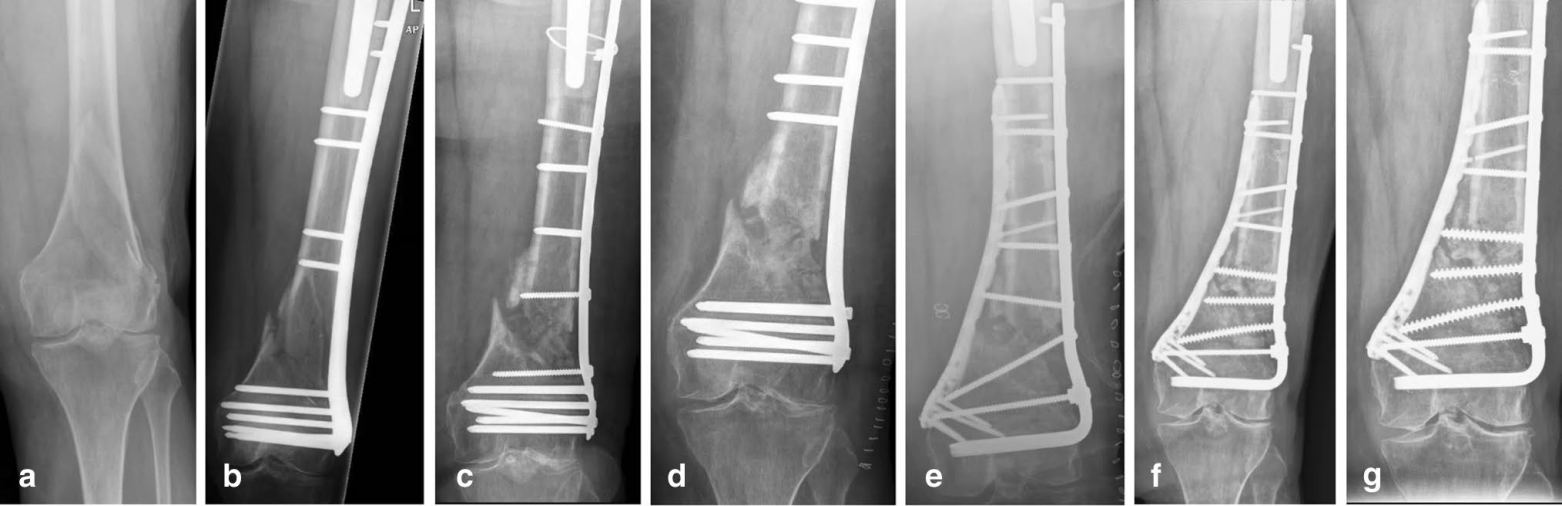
A seventy-six year old female was referred to us after several attempts to heal a comminuted extra-articular distal femur fracture (33A3) (a). Initial fracture treatment in another institution consisted of a LISS plate (b). Revision surgery with a new LISS plate and cerclage around hip prosthesis was performed 8 months after initial fracture treatment (c). Three days later a second revision surgery with a LISS plate was performed due to malposition of the previous plate (d). Six months later the patient underwent additional bone grafting surgery and was then referred to us with a persistent nonunion. At 20 months after the initial fracture we performed our index nonunion surgery with a 95-degree condylar blade plate and a Philos plate medially. In addition, autologous bone grafting was performed (e). Another bone grafting surgery with addition of three cancellous screws was performed six months later. Union was confirmed 2 months later (f). Radiographic imaging 19 months after index nonunion surgery show bone union and a well preserved knee joint (g)
